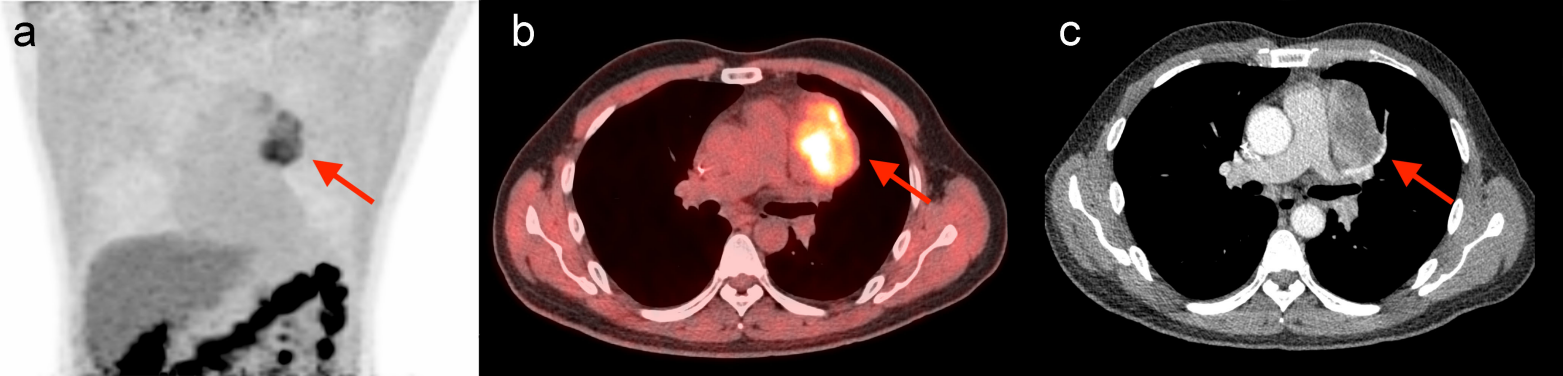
Another representative case of SD prediction. A 38-year-old man was pathologically diagnosed with SCC, and staged as IIIB (T4N2M0). The patient received six cycles of paclitaxel albumin, carboplatin, plus pembrolizumab before CIRT. The Pre-score 2 was 2.86, above the cut-off value of 0.93, and should be predicted as hypoxia. The PET/CT MIP (a) and axial fusion (b) images detected the single tumor (red arrow) with obvious ^18^F-FMISO uptake in the upper lobe of the left lung, with SUVmax of 5.26, TMR of 3.55, and HTV of 48.92 ml, indicating that the tumor was hypoxic. The patient also received CIRT with 77 Gy/22Fx. The quantitative Pre-score 1 calculated was 3.15, far above the cut-off value of -0.84, indicating a prediction of SD. Thoracic contrast-enhanced CT (c) after CIRT confirmed the tumor response as SD.
